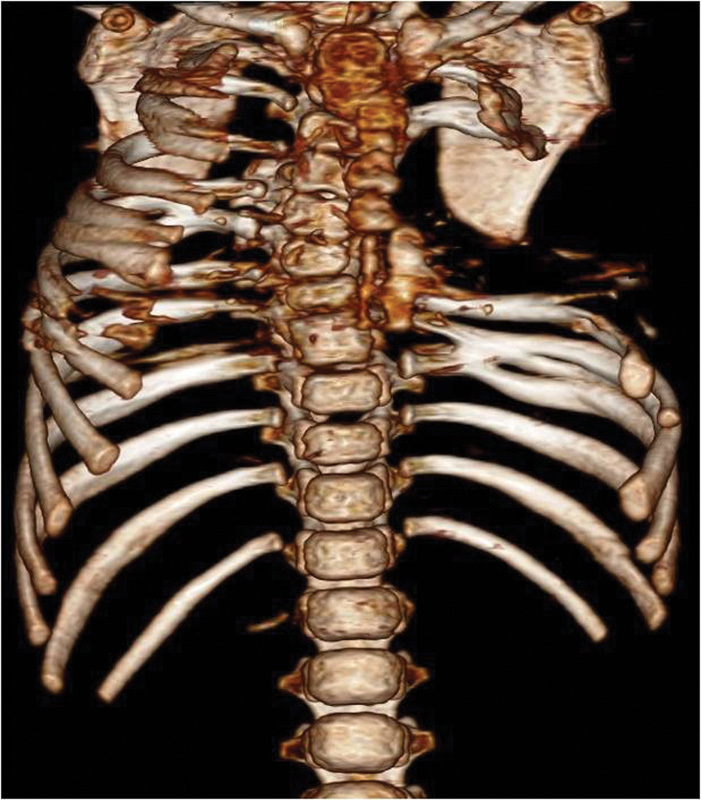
3D computed tomography (CT) reconstruction image of the chest (anterior
